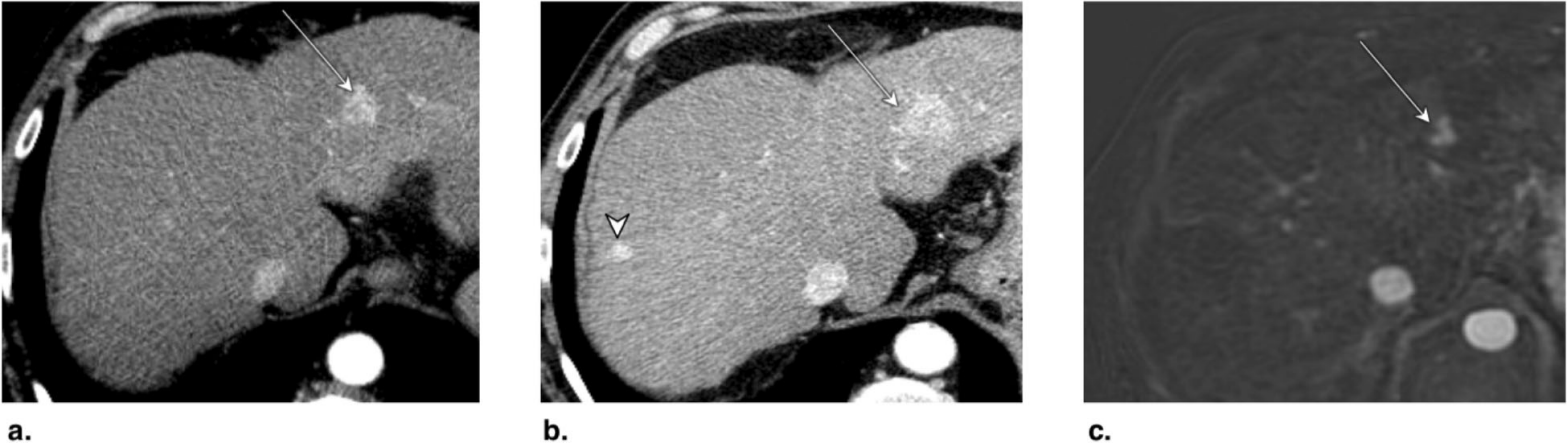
Axial CT in late arterial phase (AP) obtained (a) pretreatment: image shows nonrim arterial phase hyperenhancement (APHE) of a 2-cm LR-4 observation in segment II-III (arrow). b 2 months post percutaneous ethanol ablation (PEA): image shows interval growth (arrow) and a new LR-4 observation (arrowhead) in segment VIII. At this point, the treated lesion is categorized LR-TR viable. c Axial T1-weighted subtraction MR in late AP obtained 1 month post repeat PEA: image shows serpiginous enhancement in the location of the treated lesion possibly representing vascular fistula. The treated observation is categorized LR-TR Equivocal
